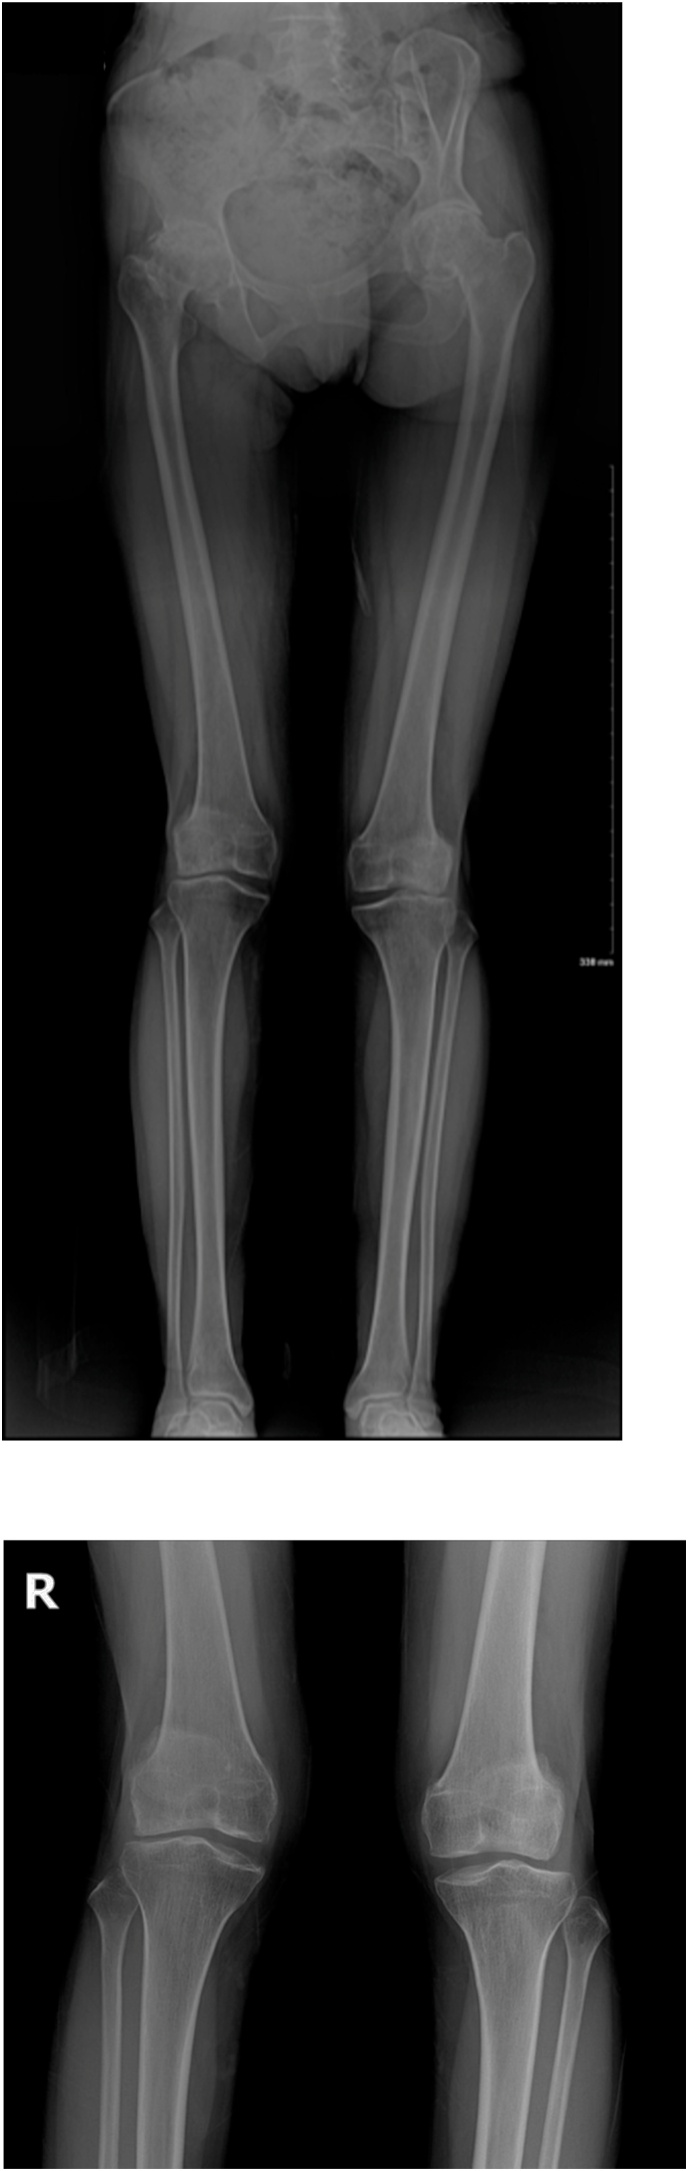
Radiography of preoperative lower extremity. A: Lower extremity scanogram showed more valgus anatomic axes (right: 12°, left 10°) than normal range (6°±3°). B: Osteoarthritis of both knees (Kellgren-Lawrence Grade III) are shown on lateral compartment and the tibial intercondylar eminence was absent in both knees. Both the medial and lateral condyles of the bilateral femurs as well as the femoral intercondylar notch were poorly developed.
